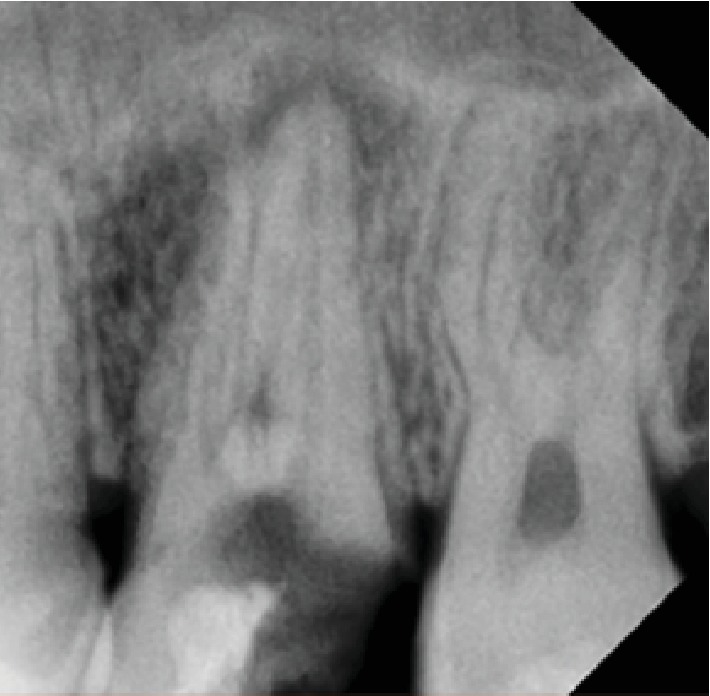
Periapical X-ray before treatment.
